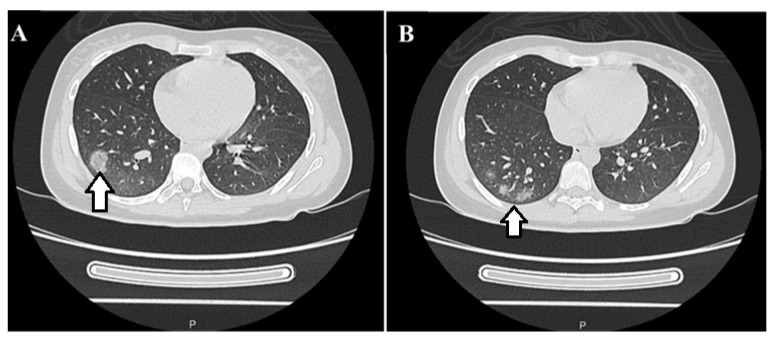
(A,B) CT scan of the chest showing ground opacities and patchy consolidation in the periphery of the right lower (white arrows) lobe before methylprednisolone.
